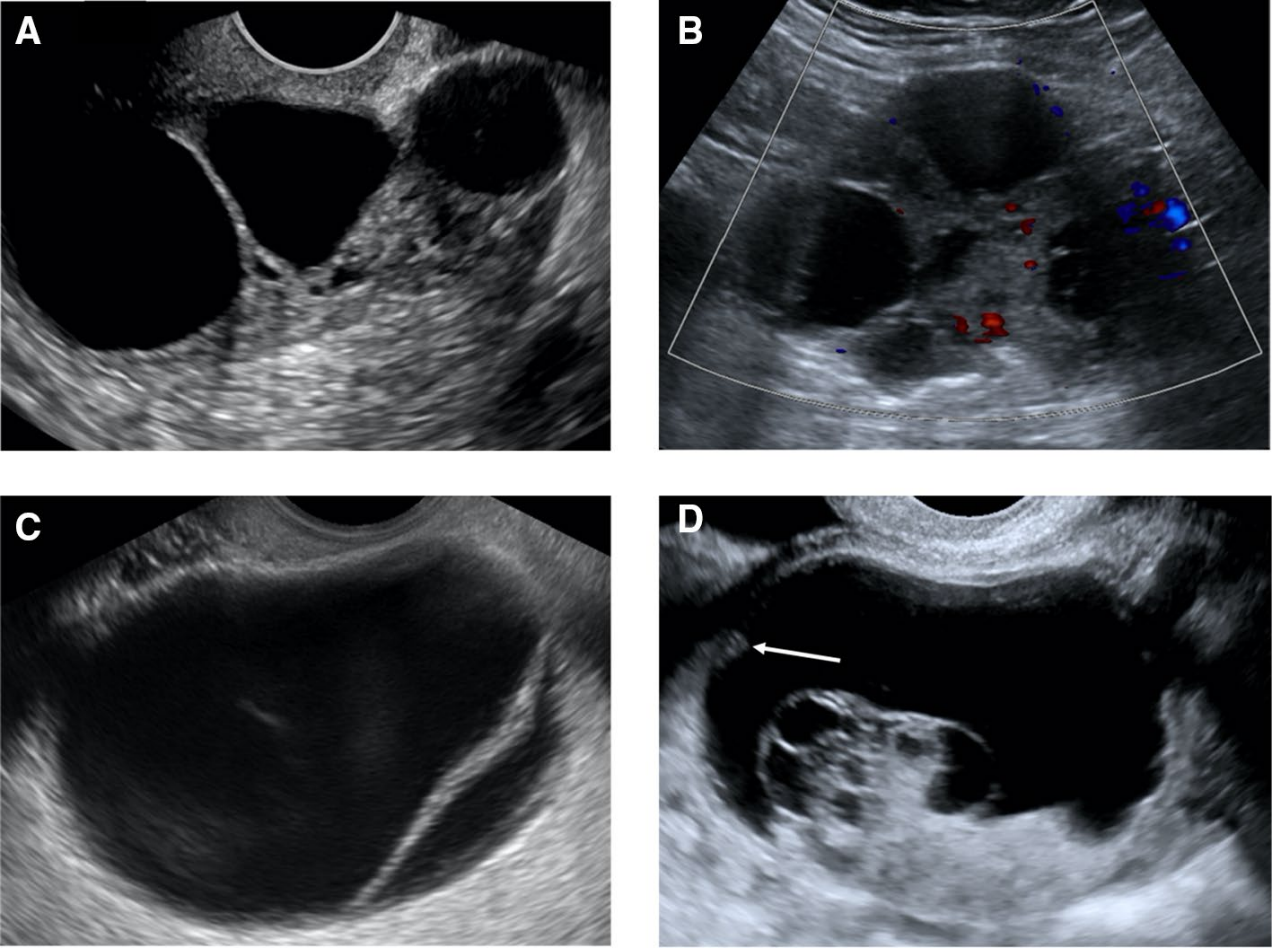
Ultrasound Examples of O-RADS Lexicon. A Grayscale image of an ovarian cystic lesion with solid component. This is an example of lesion type, which had substantial interobserver agreement. B Color Doppler image of an ovarian cystic lesion with solid component. This is an example of color score 2 (mild), which had good interobserver agreement. C Grayscale image of ovarian cyst with a smooth septation. This is an example of septation type, which had moderate interobserver agreement. D Grayscale image of a complex ovarian cystic lesion with white arrow denoting the irregular inner wall. This is an example of inner wall, which had fair interobserver agreement
